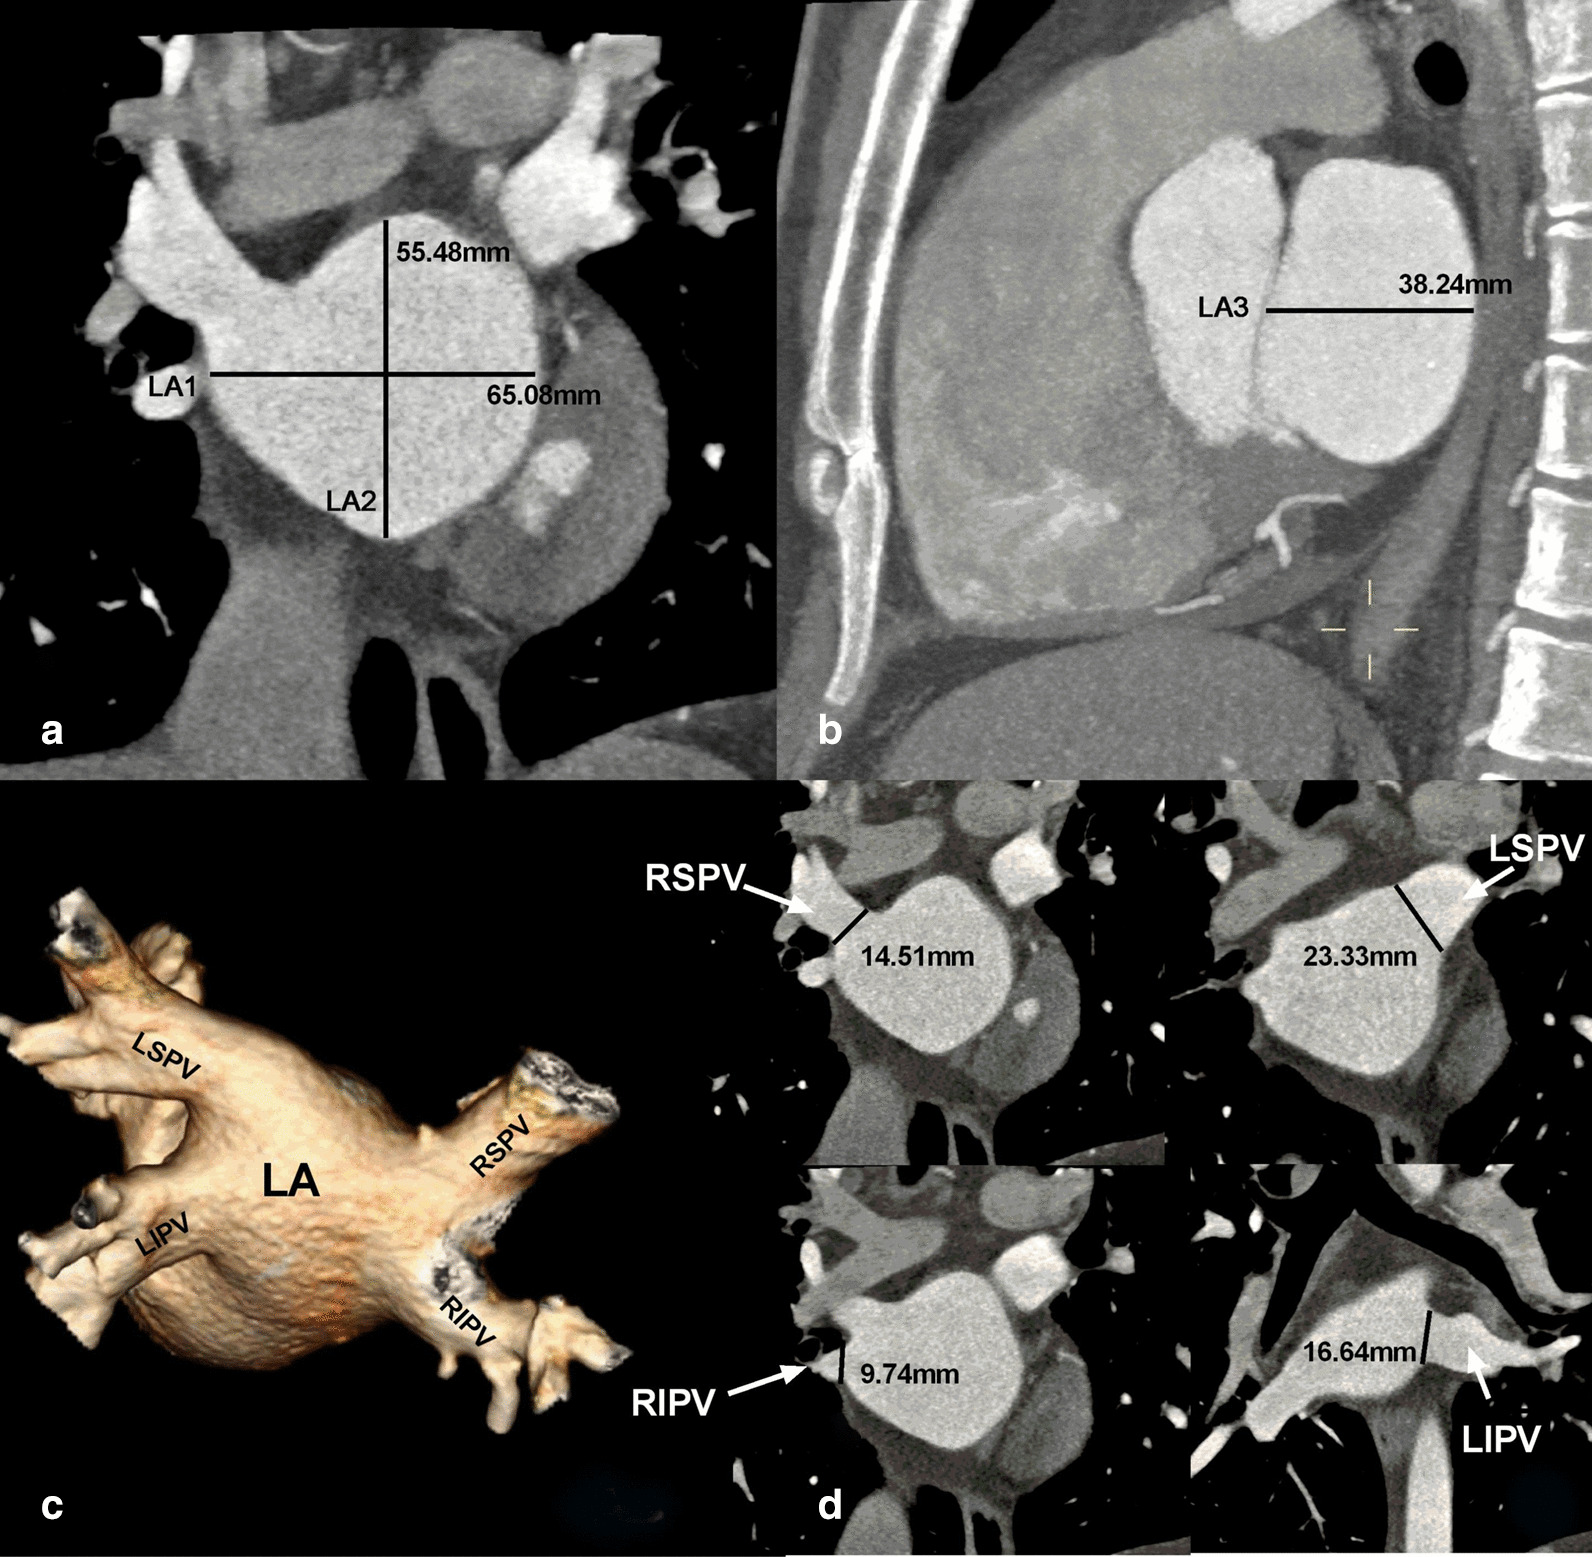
Measurement of the left atrium (LA) size and diameters of the pulmonary vein (PV) with multi-slice spiral CT. a, b Maximum diameters of the LA in coronal and sagittal views, respectively. LA1: transverse diameter; LA2: vertical diameter; LA3: anterior and posterior diameter. c Three dimensional images of the LA and four PVs. d Maximum upper and lower diameters of the four PVs measured at the coronary view. RSPV, right superior PV; RIPV, right inferior PV; LSPV, left superior PV; LIPV, left inferior PV
